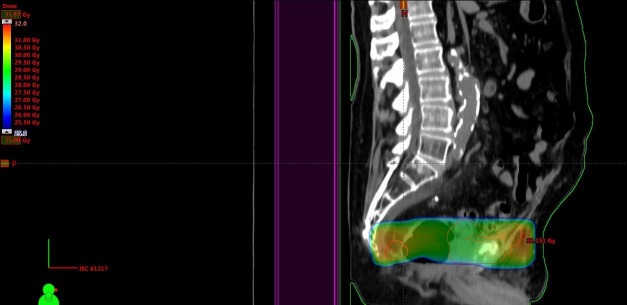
Sagittal computed tomography (CT) scan and dose distribution (course 4) Doses above 25 Gy are displayed, maximum dose 32 Gy.
